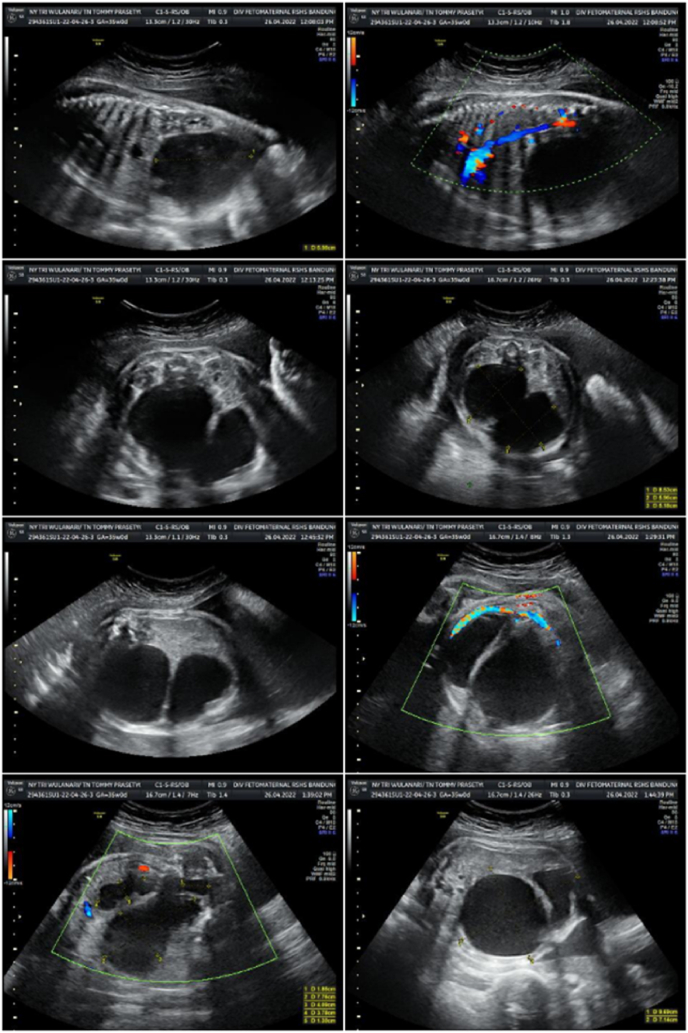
The prenatal ultrasound images at 32 weeks of gestation.
